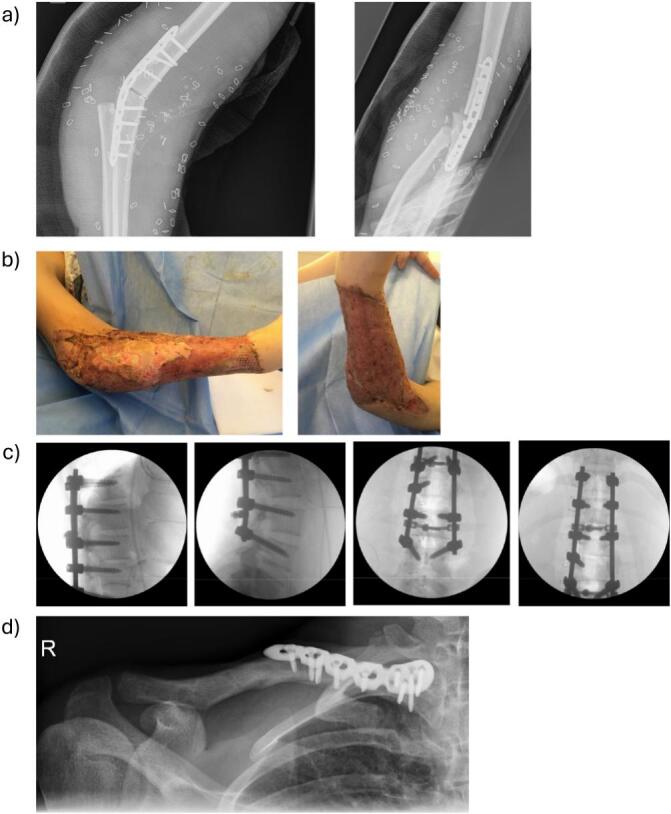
Operative treatment: a) X-ray of elbow-arthrodesis, b) clinical photography after replantation and mesh-grafting, c) fluoroscopy of dorsal instrumentation, d) X-ray of plate osteosynthesis of peri-implant clavicle fracture.
